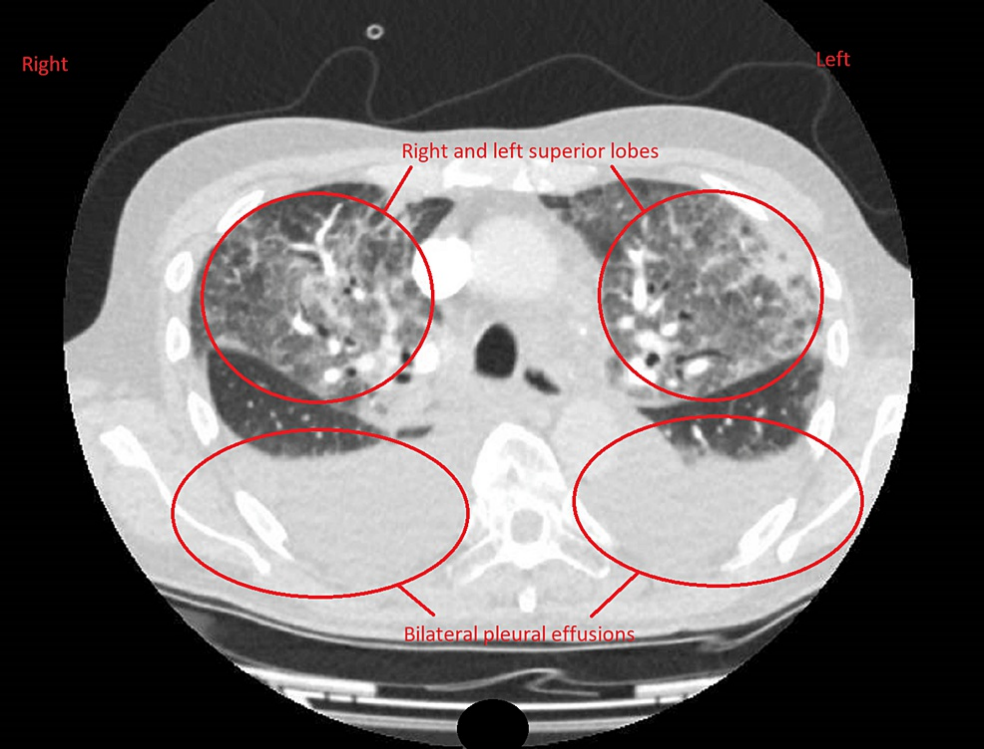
CT pulmonary angiogram axial plane CT pulmonary angiogram showing pulmonary oedema involving bilateral superior lobes and bilateral pleural effusions
